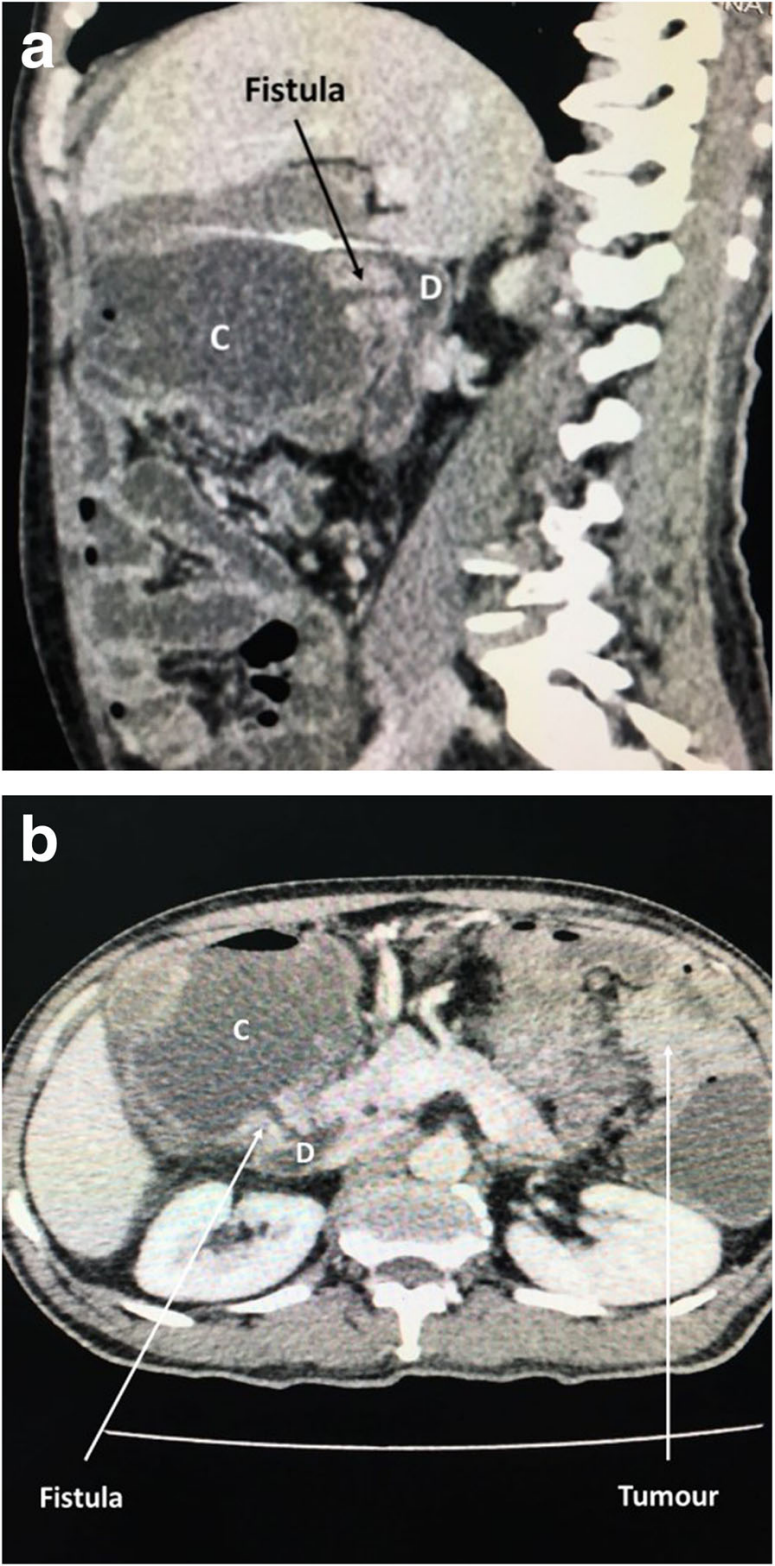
a Computed tomographic scan sagittal view demonstrating fistula between hepatic flexure (C) and duodenum (D). b Computed tomographic scan axial view demonstrating fistula between hepatic flexure (C) and duodenum (D) and distal transverse colon tumor
